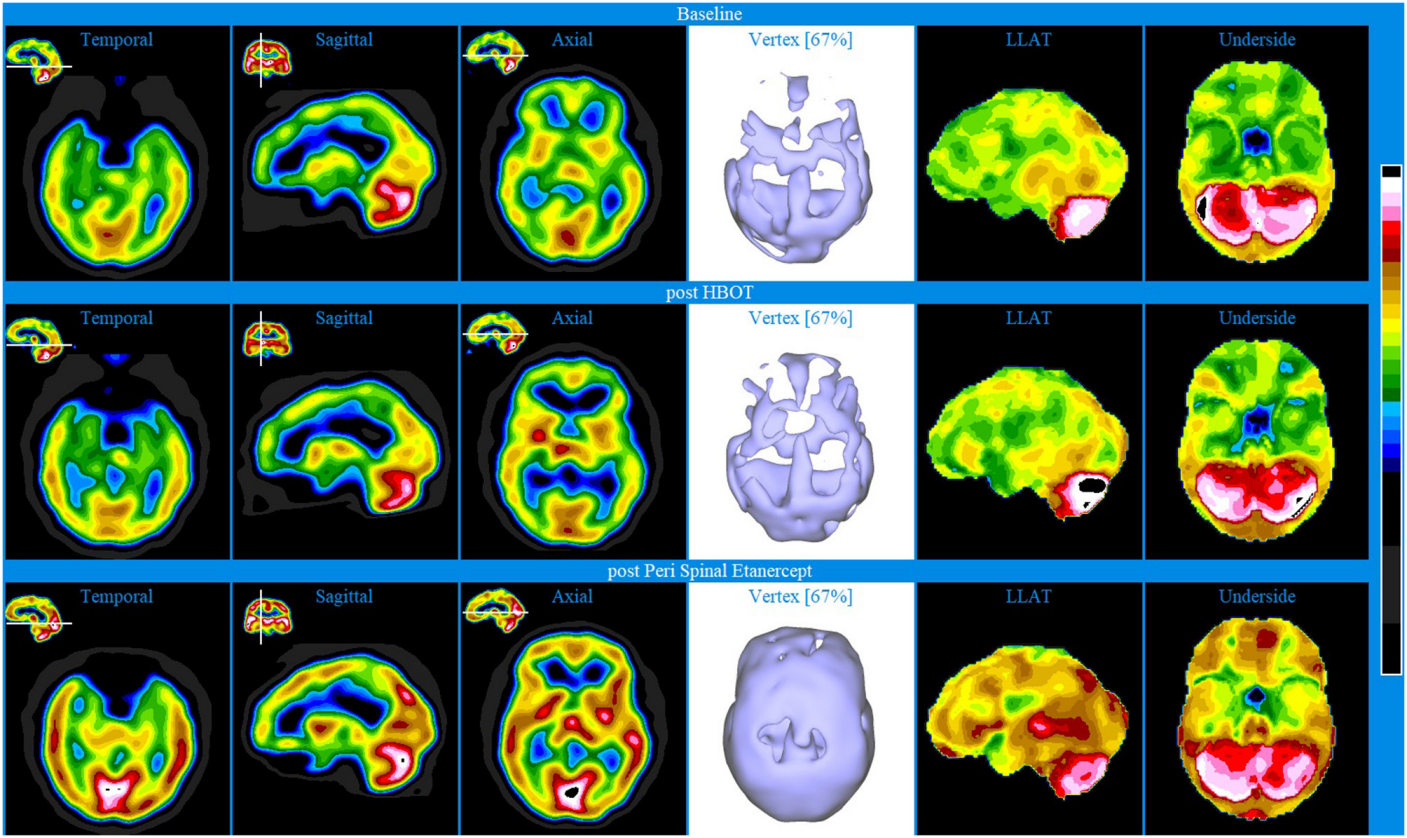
Brain SPECT images for Patient II. Top line relates to brain SPECT images at baseline, indicating extensive areas of hypofunctioning in all lobes. Middle line shows SPECT images post-HBOT treatment. Bottom line of SPECT images collected post-PSE treatment. In each row (from left to right) one temporal axial image, two orthogonal slices (sagittal and axial), one iso-surface image of the vertex and two stereotactic surface projections of the left lateral (LLAT) and underside.
